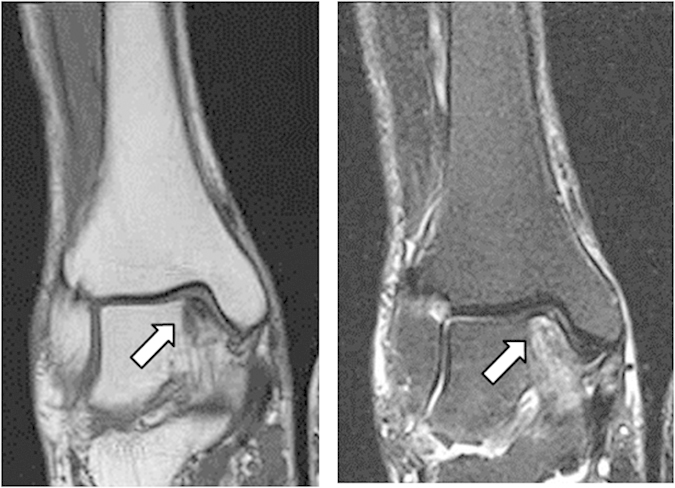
Frontal view T1W (left panel) and STIR (right panel) MR images of KL grade I OA. Although bone signal changes were observed along the ankle joint line, they were localized in the talus.
